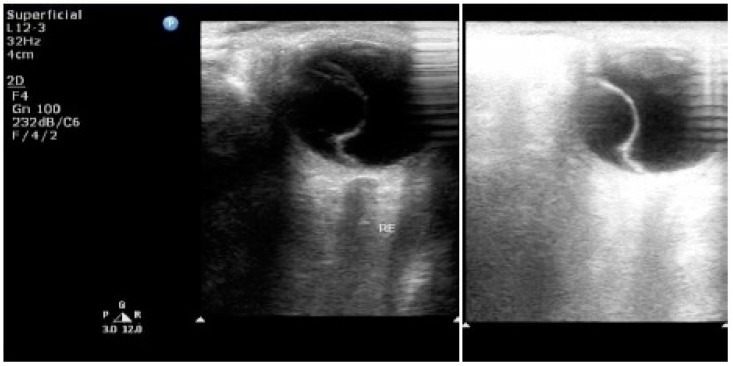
2-D ultrasonography of the right eye showing a detachment of the retina—serous variety [8]. Published with permissions of the publisher-Scholar Science Journals and International Journal of Biomedical Research. Original source: Tayade, S.; Wattamwar, A. Bilateral retinal detachment in pregnancy complicated by preeclampsia, eclampsia and placental abruption. *Int J Biomed Res*
